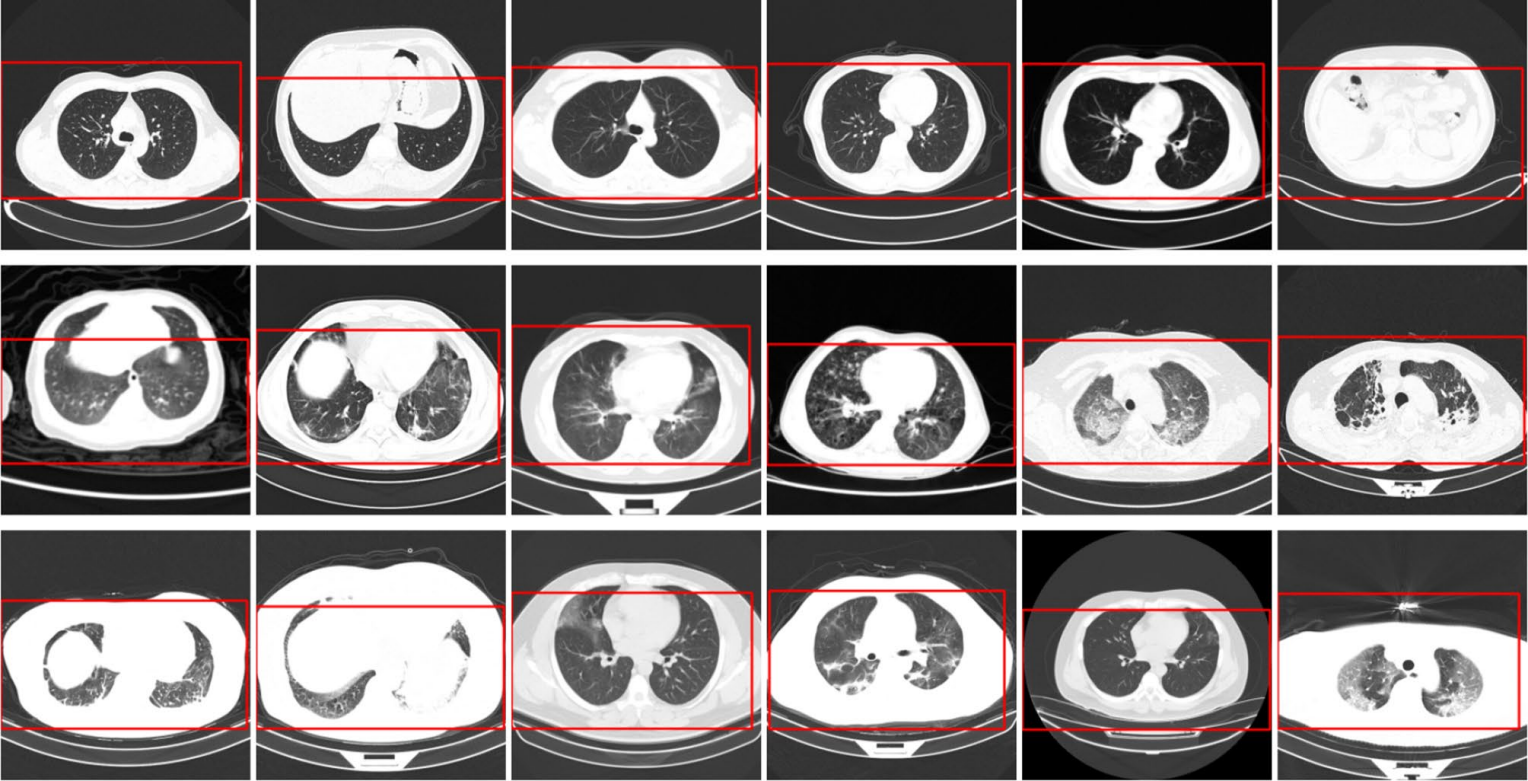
Relevant examples of CT images in COVIDx CT-2. The red frames refer to the marker frames of CT images in the dataset. The first line shows the normal controls, the second line shows the cases with CP, and the third line shows the cases with NCP infected by SARS-CoV-2.
